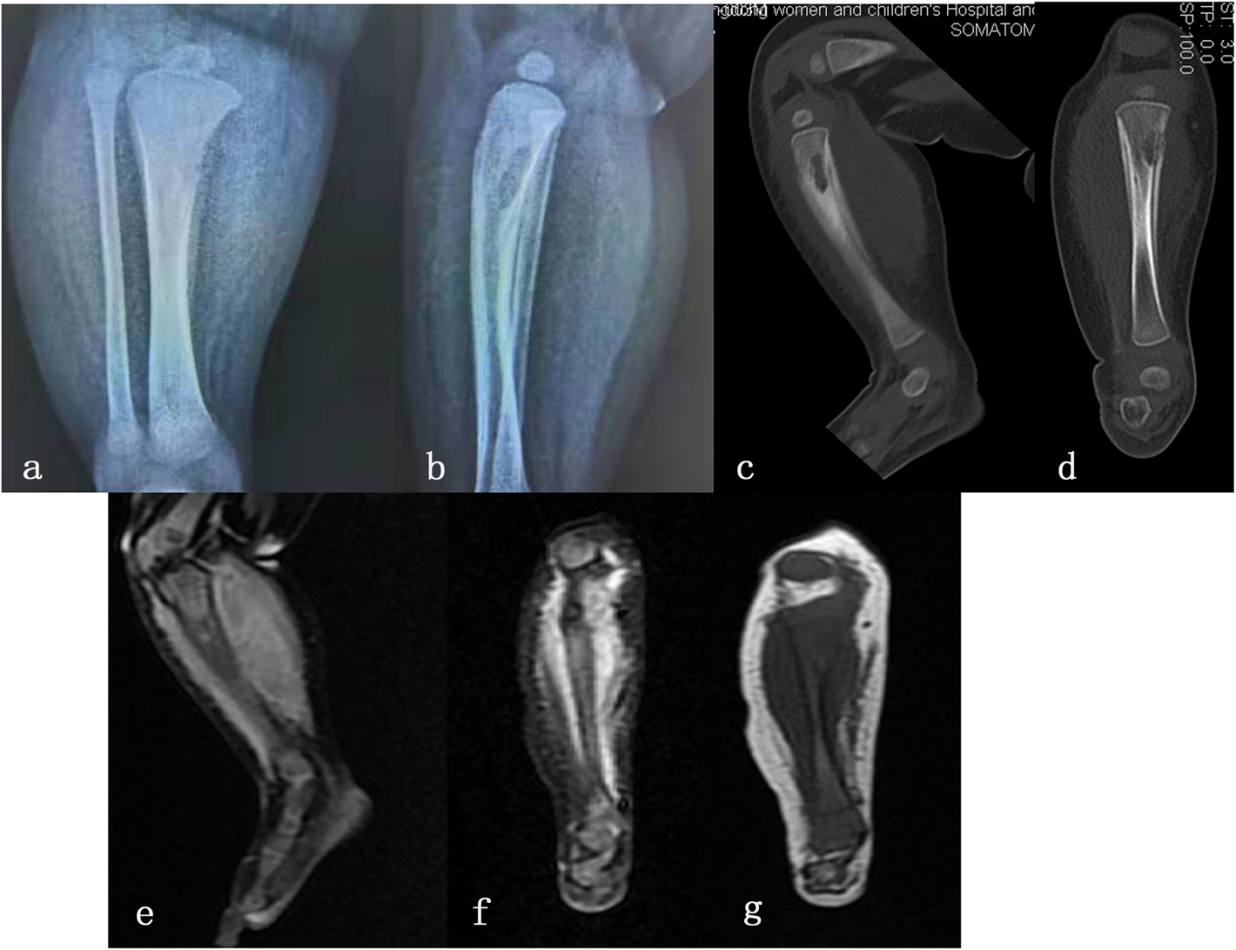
a and b Radiographs demonstrated a lytic lesion with periosteal reaction in proximal tibia in a 2 months old patient. c and d CT coronal and sagittal plane revealed a moth-eaten, ice melting sign, irregular erosions on her tibial metaphysis. e-g T1-weighted MRI confirms the processes of hypointense under the proximal tibial physis corresponding to hyperintensity on T2-weighted images with cortical breach and adjacent soft-tissue abscess
